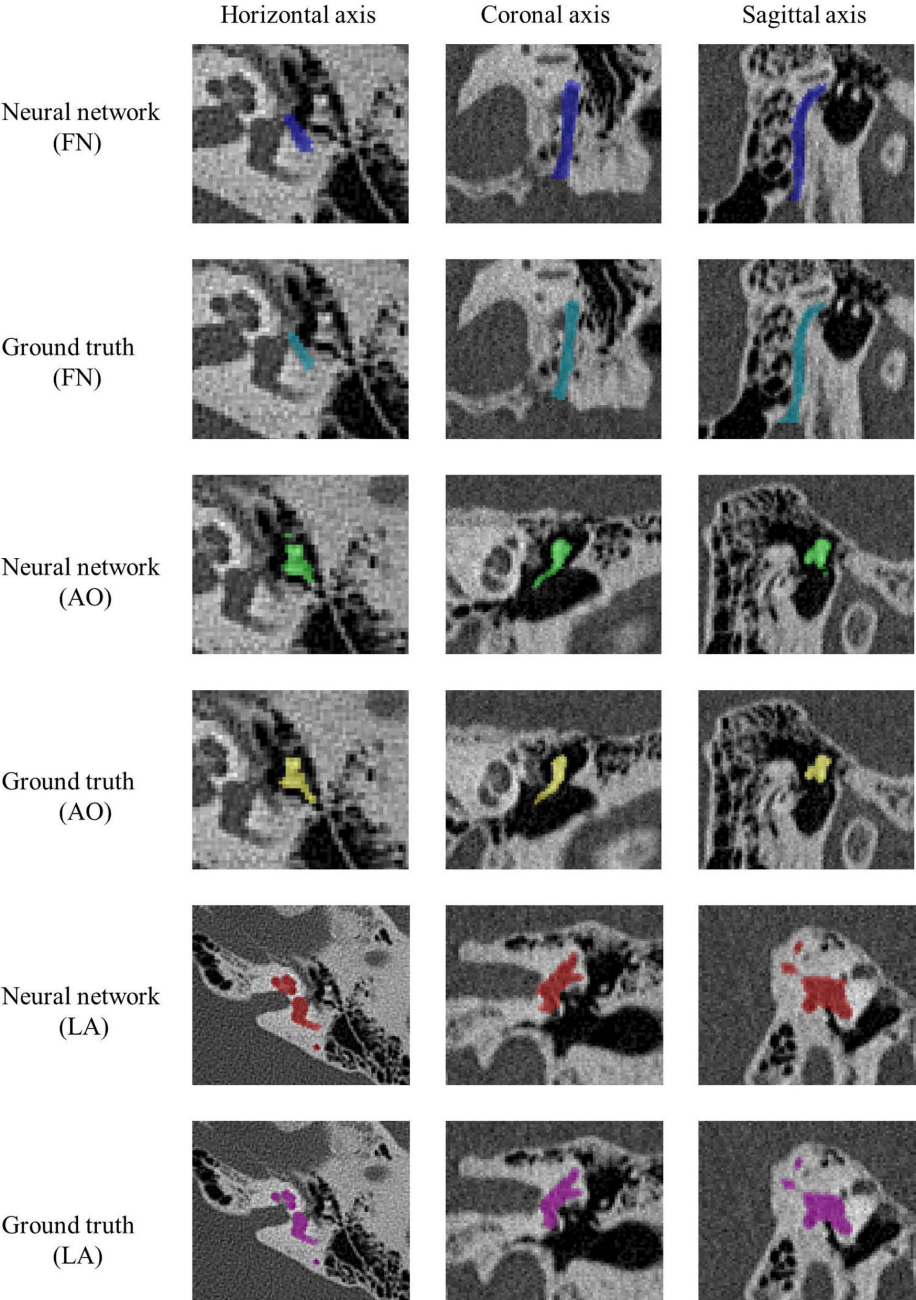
The masks of important structures generated by two methods in normal adult temporal bone CT images. Blue, green, and red labels denote the automatic extraction of FN, AO, and LA, respectively. Turquoise, yellow, and magenta labels represent the manual segmentation of FN, AO, and LA, respectively. CT, computed tomography; FN, facial nerve; AO, auditory ossicle; LA, labyrinth
